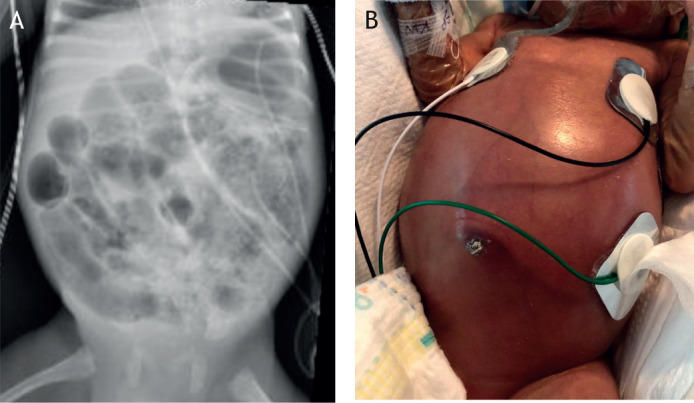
Clinical features of NEC in a child. (A) Abdominal x-ray of a premature baby showing extensive pneumatosis intestinalis. (B) Clinical appearance of a patient with NEC. Image credit: M.L.K.
